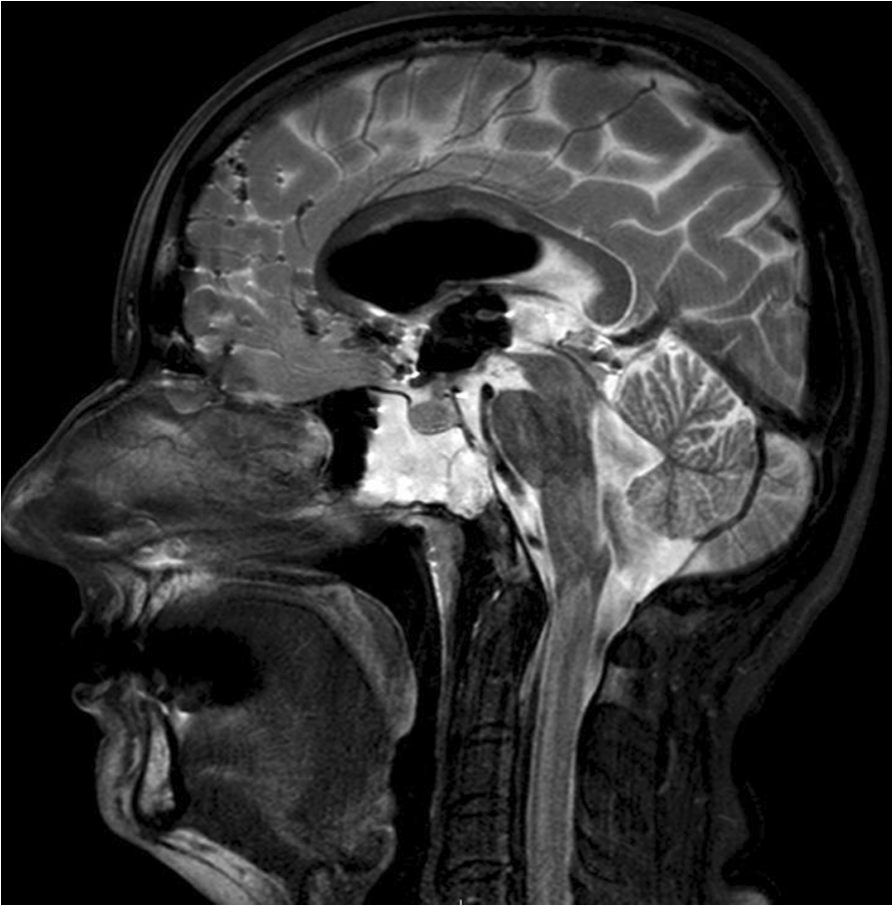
T2-weighted sagittal images showing erosion of the posterior wall of the clivus and hyperintensity suggestive of cerebral spinal fluid, with a fluid level in the sphenoid sinus. Note also, the presence of pneumocephalus with air in the subarachnoid spaces and the ventricle.
